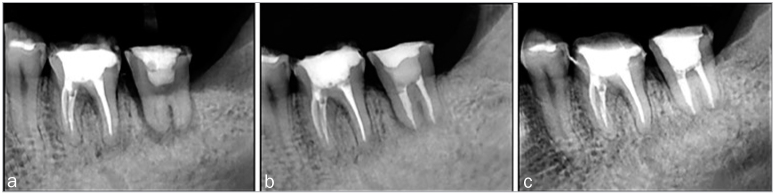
Conventional intraoral radiography. (a) 1 month after autotransplantation; (b) 6 months after autotransplantation; and (c) 12 months after autotransplantation.
